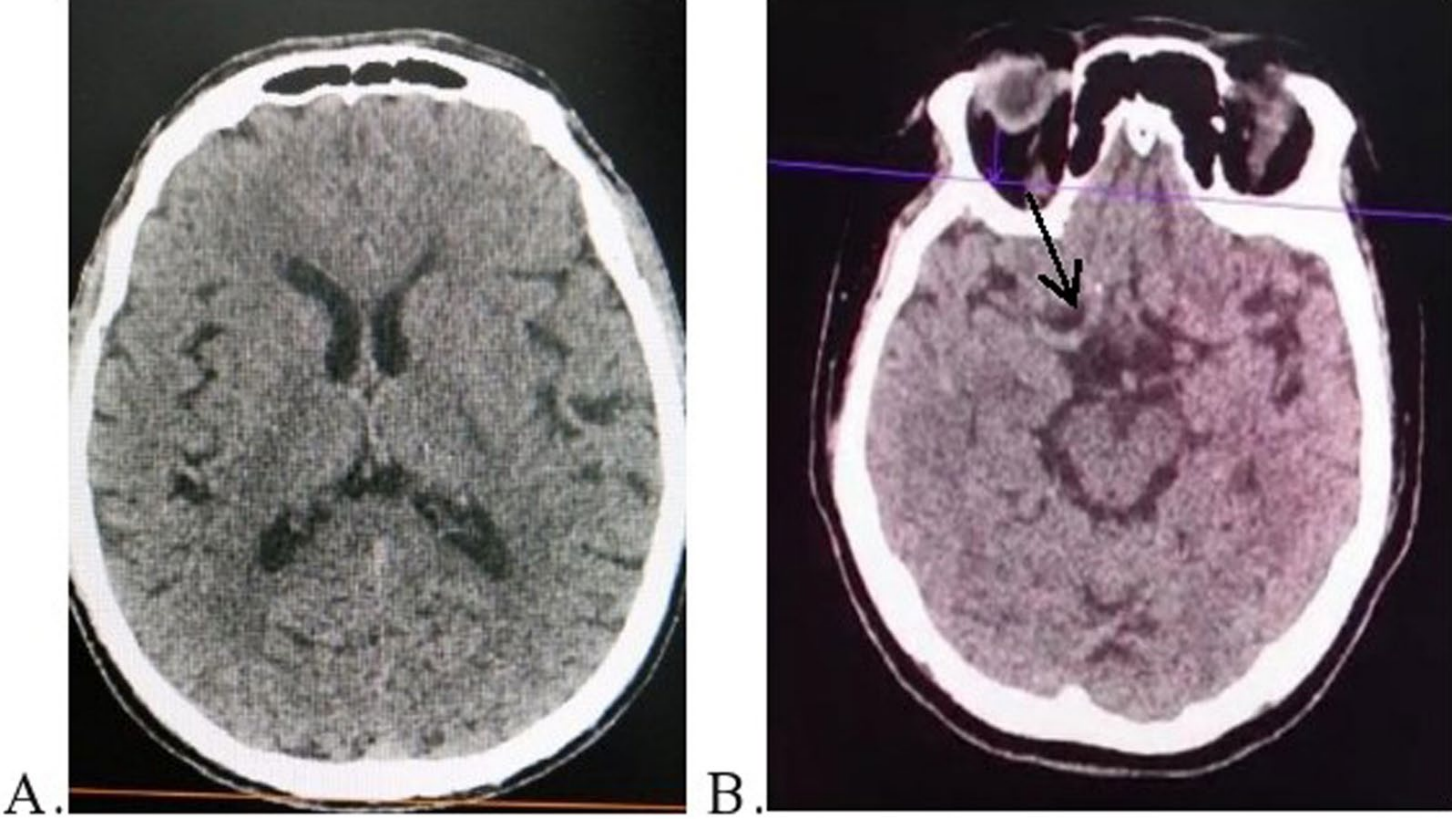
Imaging of ischemic stroke patients with COVID-19. A CT brain from case 1, showing hypodensities in left ganglia basal, insula and internal capsule. B Case 2, hypodensities were not found, MCA dense sign were prominent (black arrow). *MCA* middle cerebral artery
